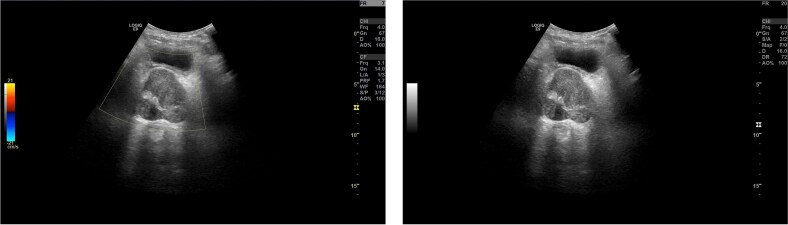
Prostate ultrasound image (transabdominal). The cross-section of the prostate is approximately 4.8 × 5.1 × 4.5 cm in size. The peripheral contour is still clear, and the shape is not regular. Multiple strong echo light spots can be seen inside, and one of them is approximately 0.3 × 0.2 cm in size.
